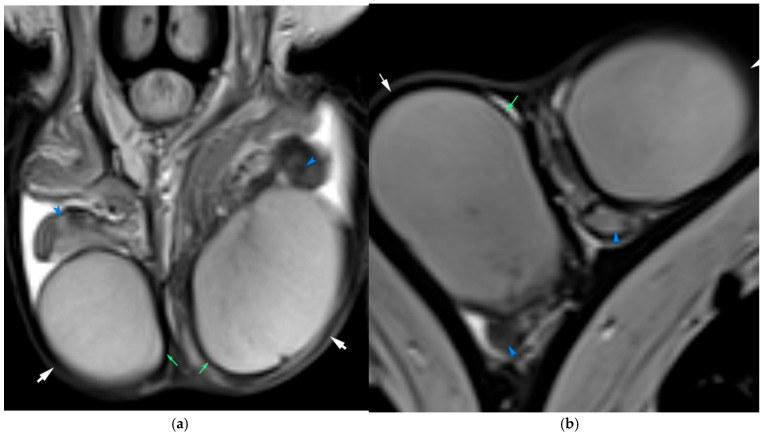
Normal scrotal anatomy on T2-weighted MRI. (a) Coronal and (b) sagittal images. Green arrows indicate the tunica albuginea; white arrows outline the scrotal wall. The epididymides are depicted by blue arrowheads. A small bilateral hydrocele is present, regarded as a normal incidental finding.
